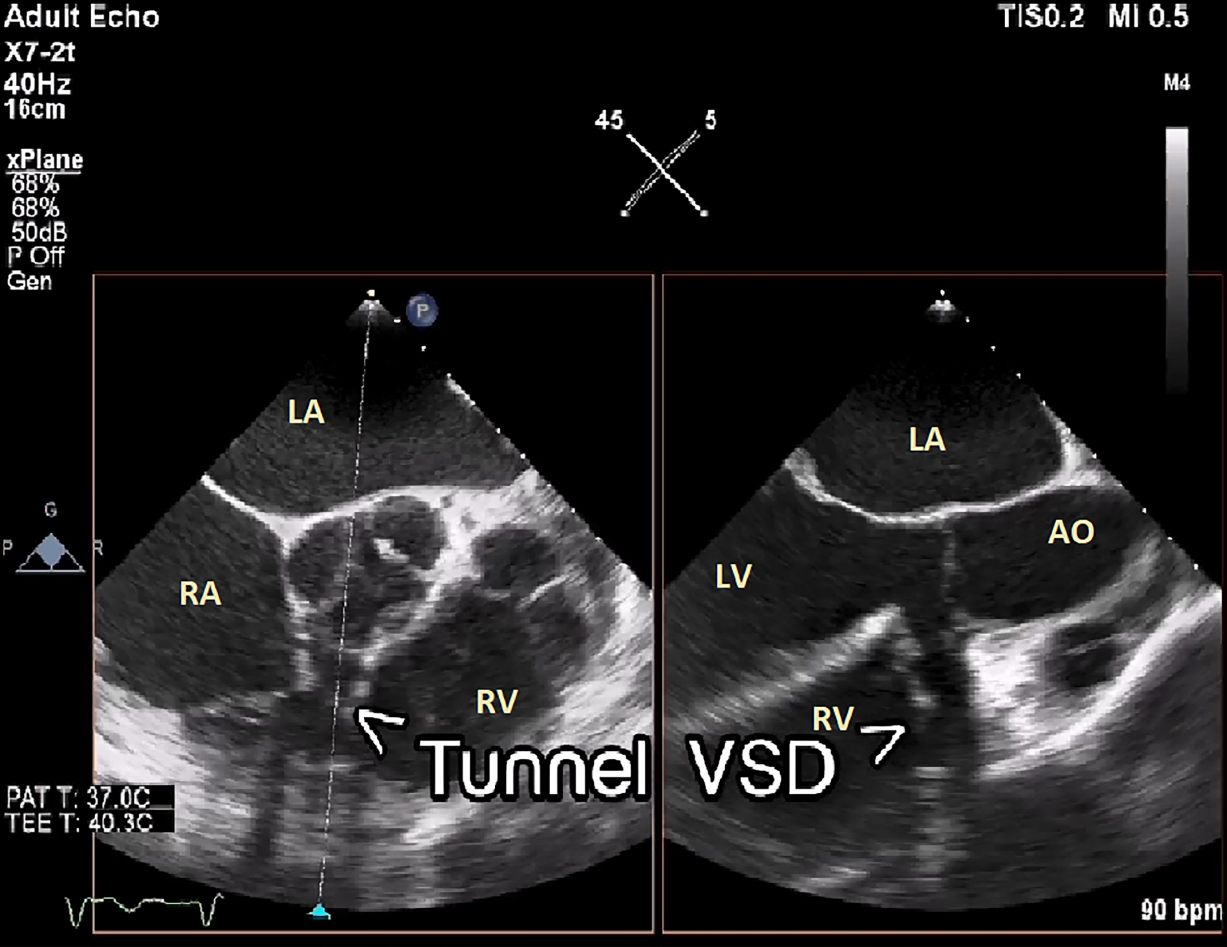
Transesophageal echocardiography at midesophageal level using the biplane method shows a tunnel shape VSD. AO, ascending aorta; LA, left atrium; LV, left ventricle; RA, right atrium; RV, right ventricle; VSD, ventricular septal defect.
